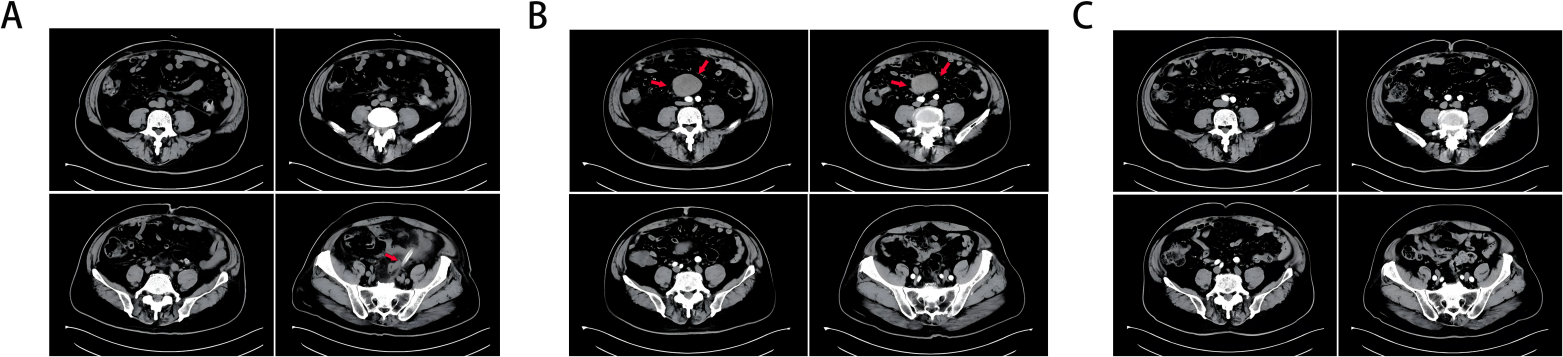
(A) CT taken 1 day after colon cancer surgery, and an arrow marks the drainage tube. (B) A retroperitoneal tumor (marked with an arrow) was found on intensive CT 1 year after colon cancer surgery, and there was vascular nourishment in the tumor. (C) Abdominal CT 3 years after resection of colon cancer and 2 years after resection of invasive fibroids, and no obvious abnormalities were found.
